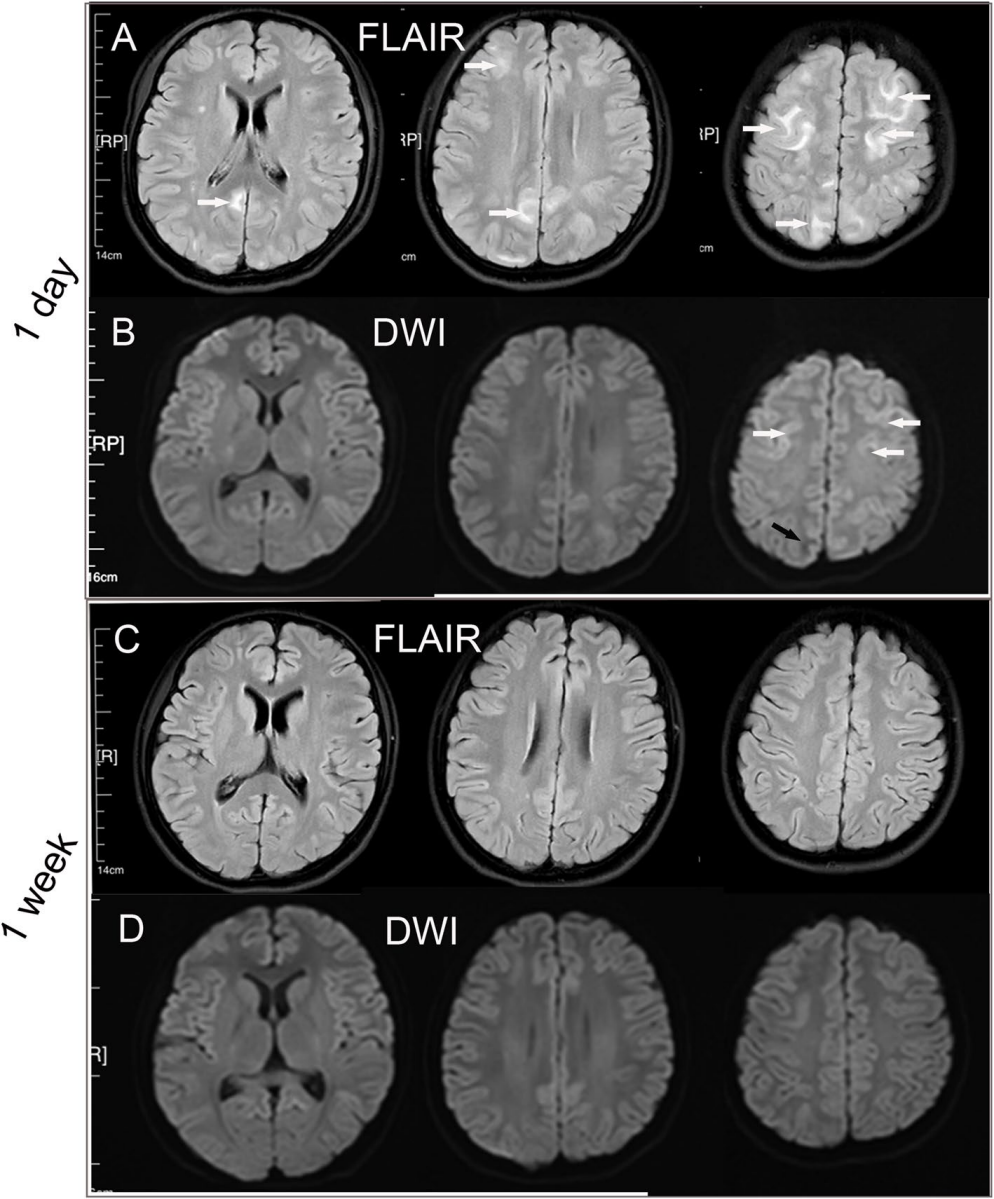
Initial and follow-up brain MRI of the patient. T2-FLAIR images obtained 1 day after the patient’s seizure show high-intensity signals in the bilateral frontal, parietal and occipital lobes (A, white arrow). DWI scans at 1 day show slightly hyperintense signals in the bilateral frontal cortex (B, white arrow) and hypointense signals in the right occipital lobe (B, black arrow). Follow-up T2-FLAIR (C) and DWI scans (D) at 1 week show resolution of the high-signal lesions
